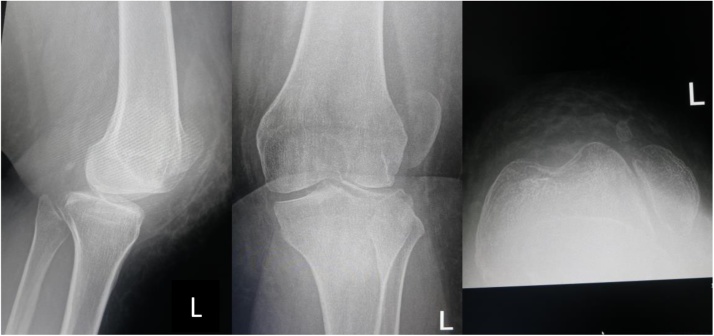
X ray AP, lateral and skyline view radiograph show the lateral patellar dislocation.
